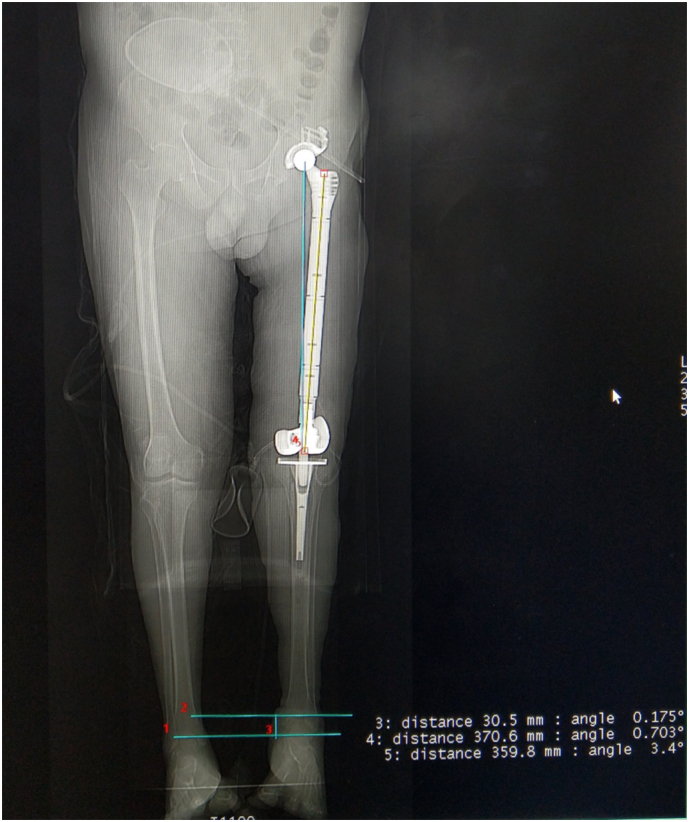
Postoperative X-ray image of the patient presented good structural integrity and anatomical resemblance with a <30 mm length discrepancy between the left and right leg.
